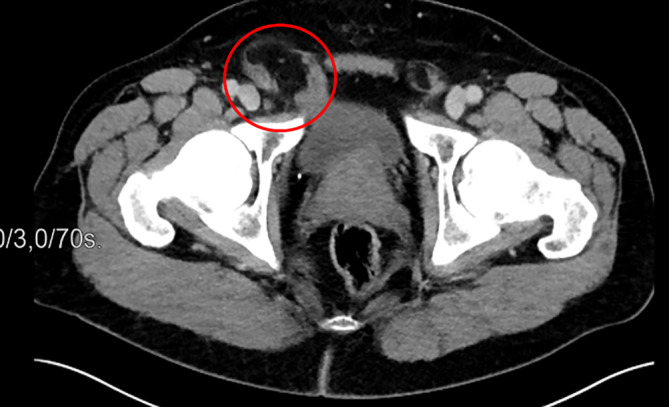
CT – Transversalscan (*roter Kreis*: umfährt die Ausdehnung der Inguinalhernie rechts mit Harnblasendivertikel im Bruchsack)
